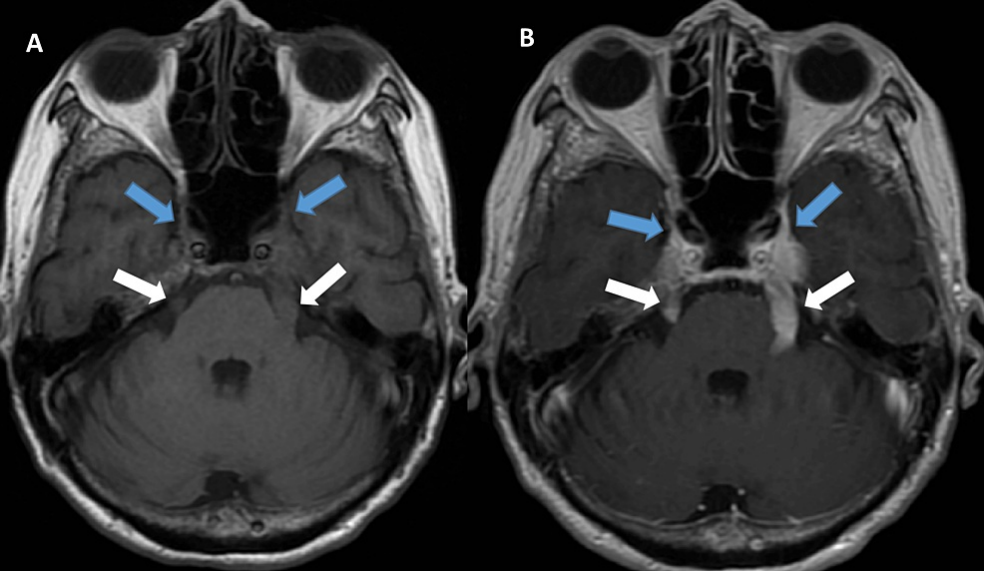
(A) MRI of the brain without contrast showing hypertrophied bilateral trigeminal (white arrows) and facial nerves (blue arrows). (B) MRI of the brain with contrast showing significant enhancement of bilateral trigeminal (white arrows) and facial nerves (blue arrows). MRI: magnetic resonance imaging.
